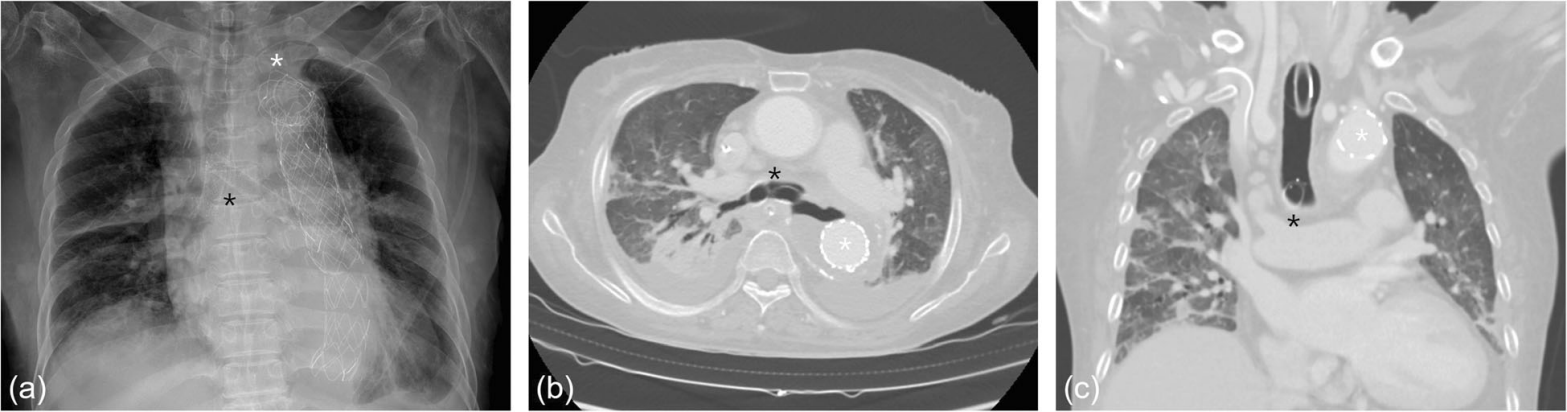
(a) Endobronchial stents (Nitinol Stent, right 10 × 40 mm and left 10 × 60 mm) are inserted in the both main bronchi. (b, c) Computed tomography of the chest after TEVAR (white start) and endobronchial stenting (black star)
